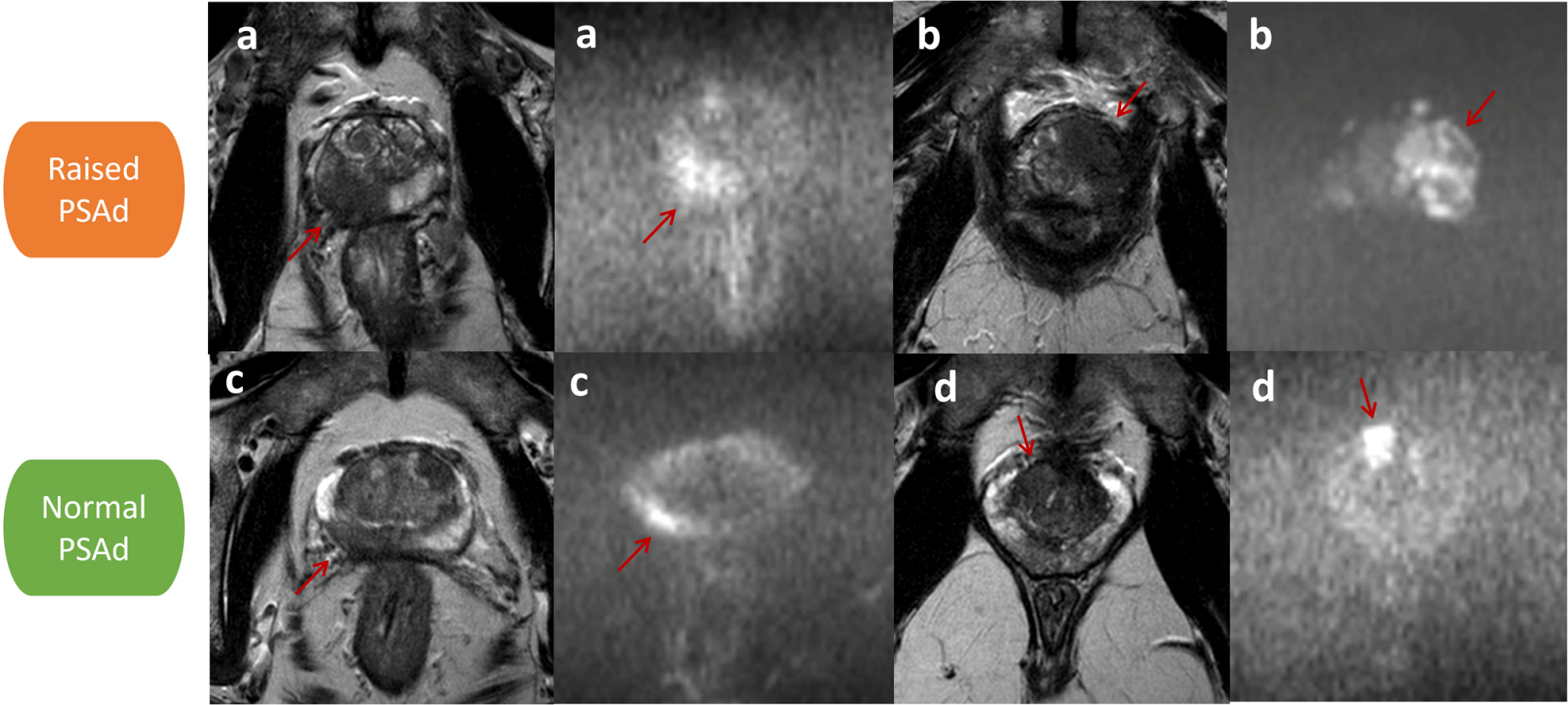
Examples of screening MRI-true-positive scans in men with raised (top) and normal (bottom) PSAd—paired T2-weighted imaging (T2WI) (left) and b2000 DWI (right) for each participant (a–d): a 72-year-old male with right peripheral zone (PZ) Gleason 4 + 5 tumour (arrow) with PSA 4.97 ng/mL and PSAd 0.18 ng/mL^2^; b 72-year-old male with left transitional zone (TZ)/PZ Gleason 4 + 5 tumour (arrow) with PSA 43.5 ng/mL and PSAd 0.75 ng/mL^2^; c 70-year-old male with right PZ Gleason 3 + 4 tumour (arrow) with PSA 1.84 ng/mL and PSAd 0.05 ng/mL^2^; and (d) 52-year-old male with right TZ Gleason 3 + 4 tumour (arrow) with PSA 2.42 ng/mL and PSAd 0.10 ng/mL^2^
